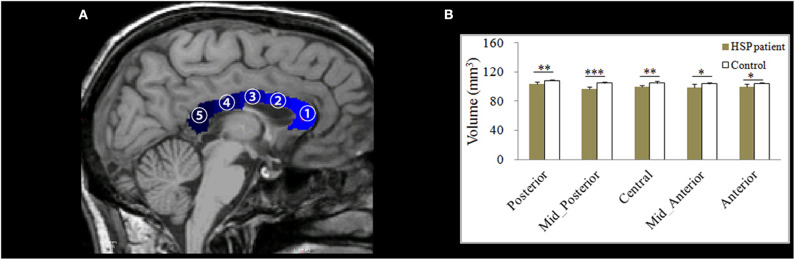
(A) An example of the division of the corpus callosum (shown in blue) into five segments on a T1-weighted image. (B) Differences in the mean volumes of the five subdivisions of the corpus callosum. ^*^*p* < 0.05; ^^*p* < 0.01; ^*^*p* < 0.001.
